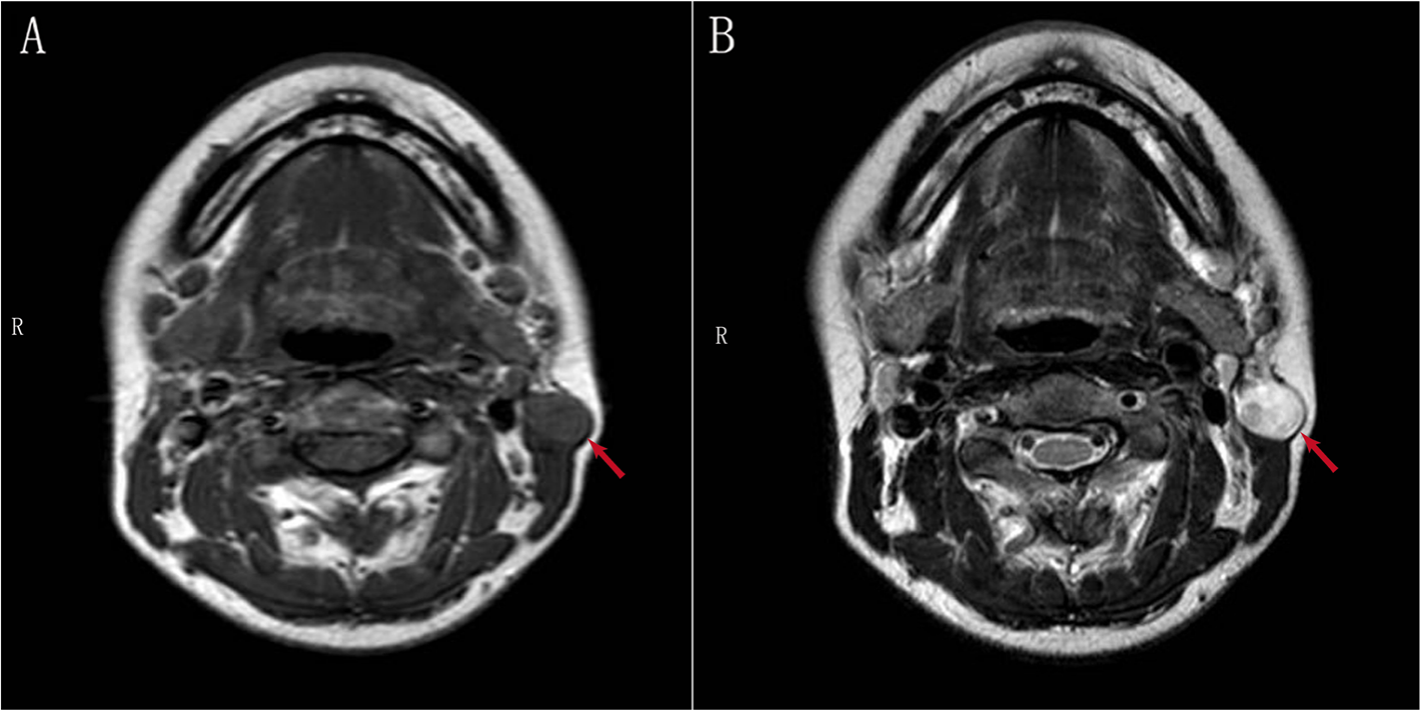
MRI revealed a high possibility of schwannoma:a subcutaneous nodule in left neck with intermediate T1 (a) and T2 signal (b) as well as clear margin (red arrow). The mass presented inhomogeneous intermediate signal at T2 similar with Antoni A as well as Antoni B area (b)
